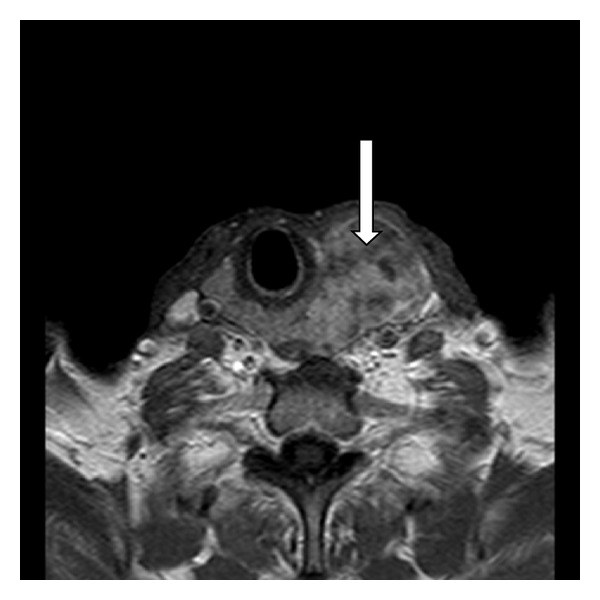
Magnetic resonance imaging scan showing heterogeneous mass in relation to the left thyroid lobe marked with an arrow.
